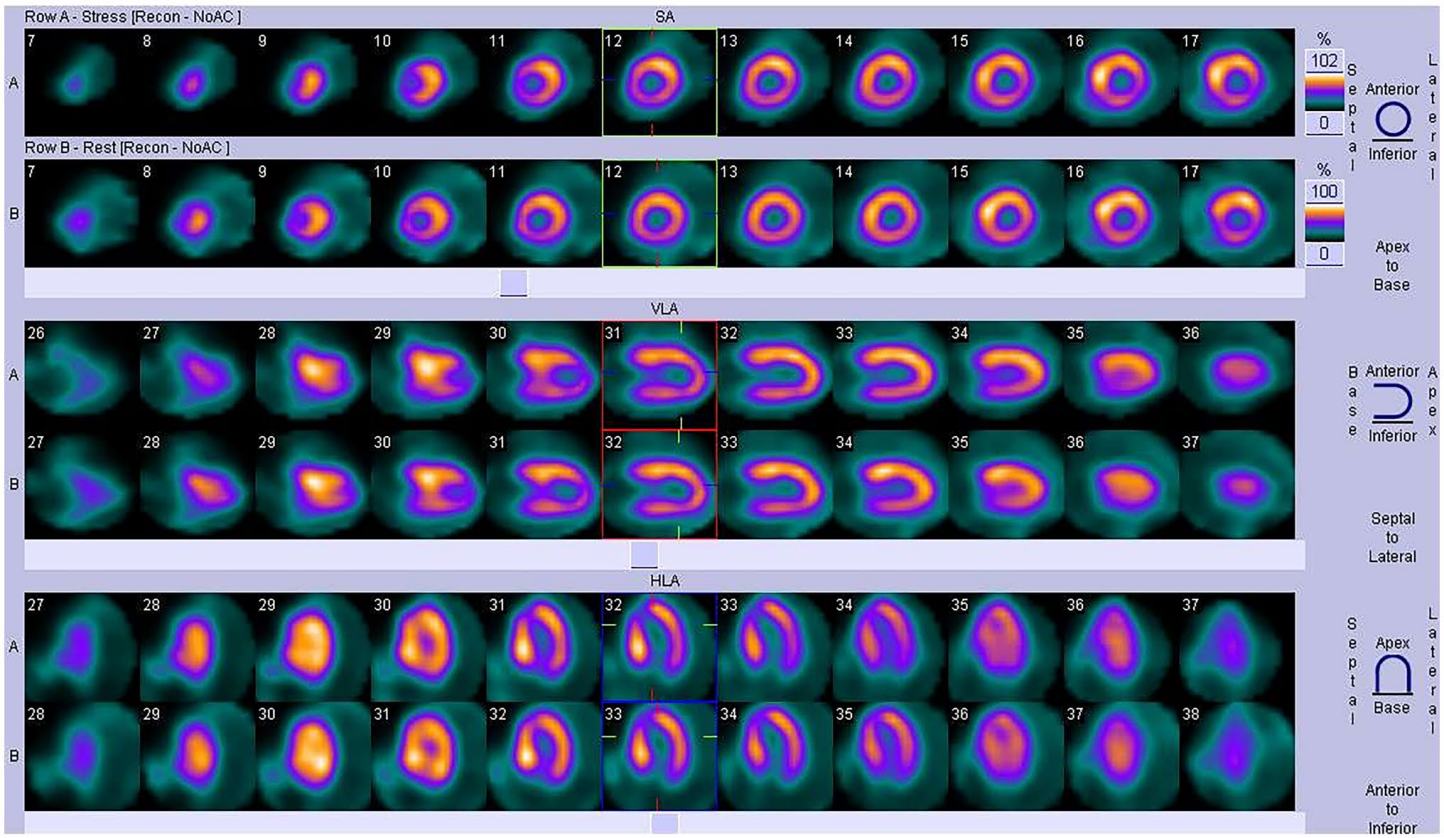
SPECT imaging: patient with septal and left ventricular posterior wall hypertrophy. Fixed perfusion defects were detected during exercise in the basal segments of septum and in the left ventricular inferior wall. Perfusion defects not resolved at rest
